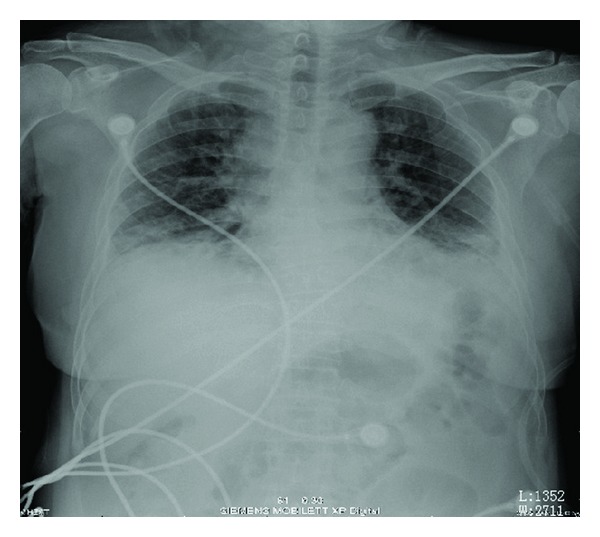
Chest radiograph with remarkable reduction of lung volume as well as increased lung markings.
